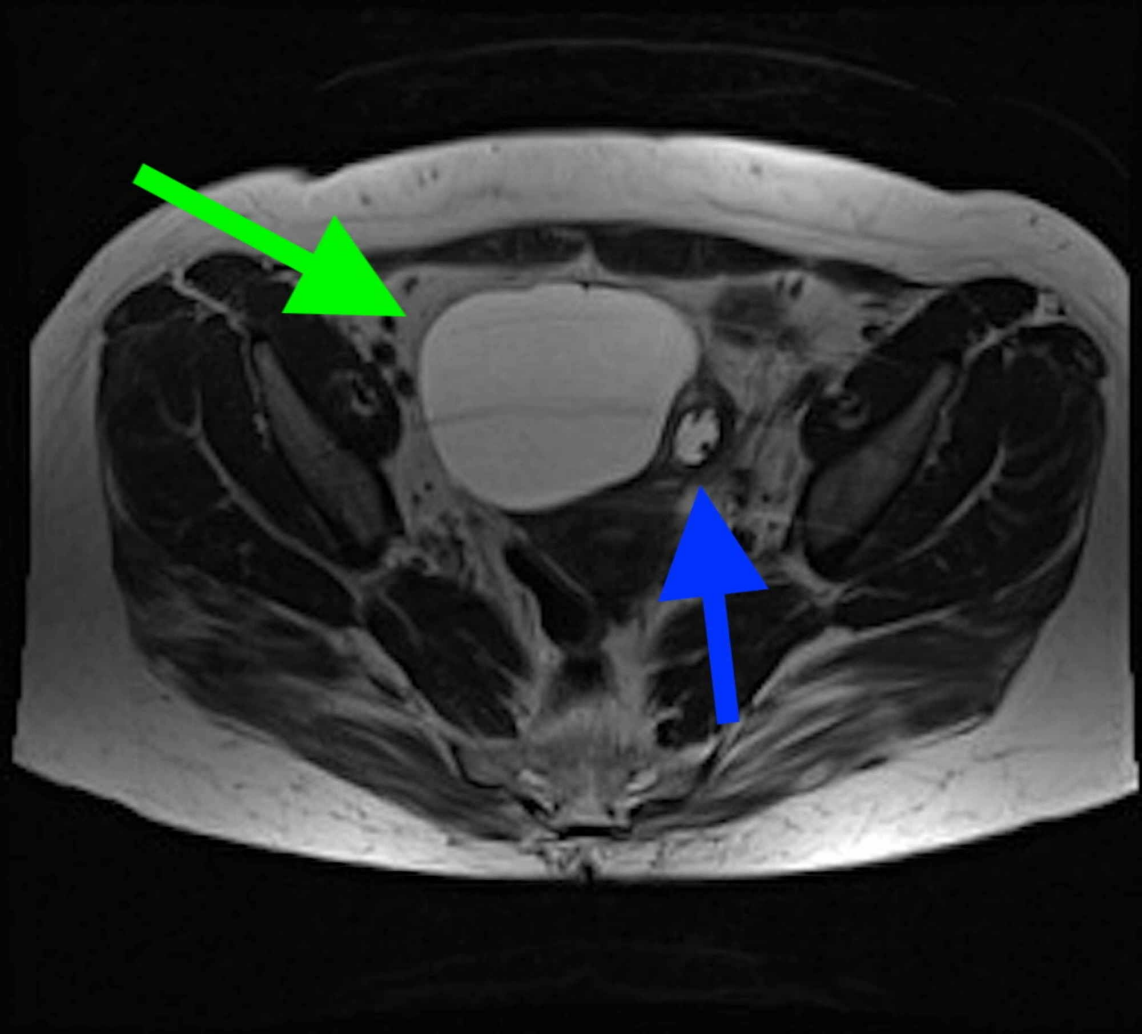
MRI transverse plane view of ovarian cyst (green arrow) causing mass effect on bladder. Nabothian cysts (blue arrow) also noted.
